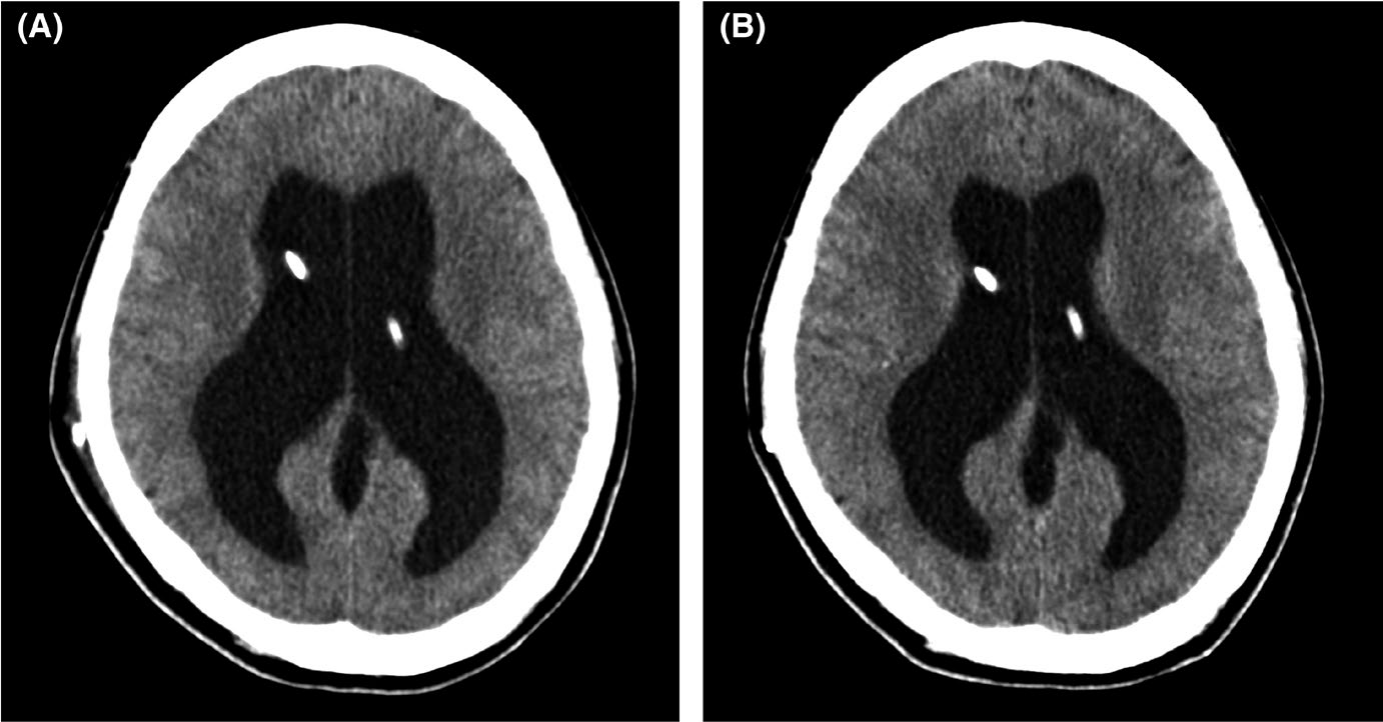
Cranial computed tomography demonstrating enlarged bilateral ventricles (A) compared to those 3 years ago (B) due to hydrocephalus and a ventriculoperitoneal catheter placed in the bilateral ventricles
